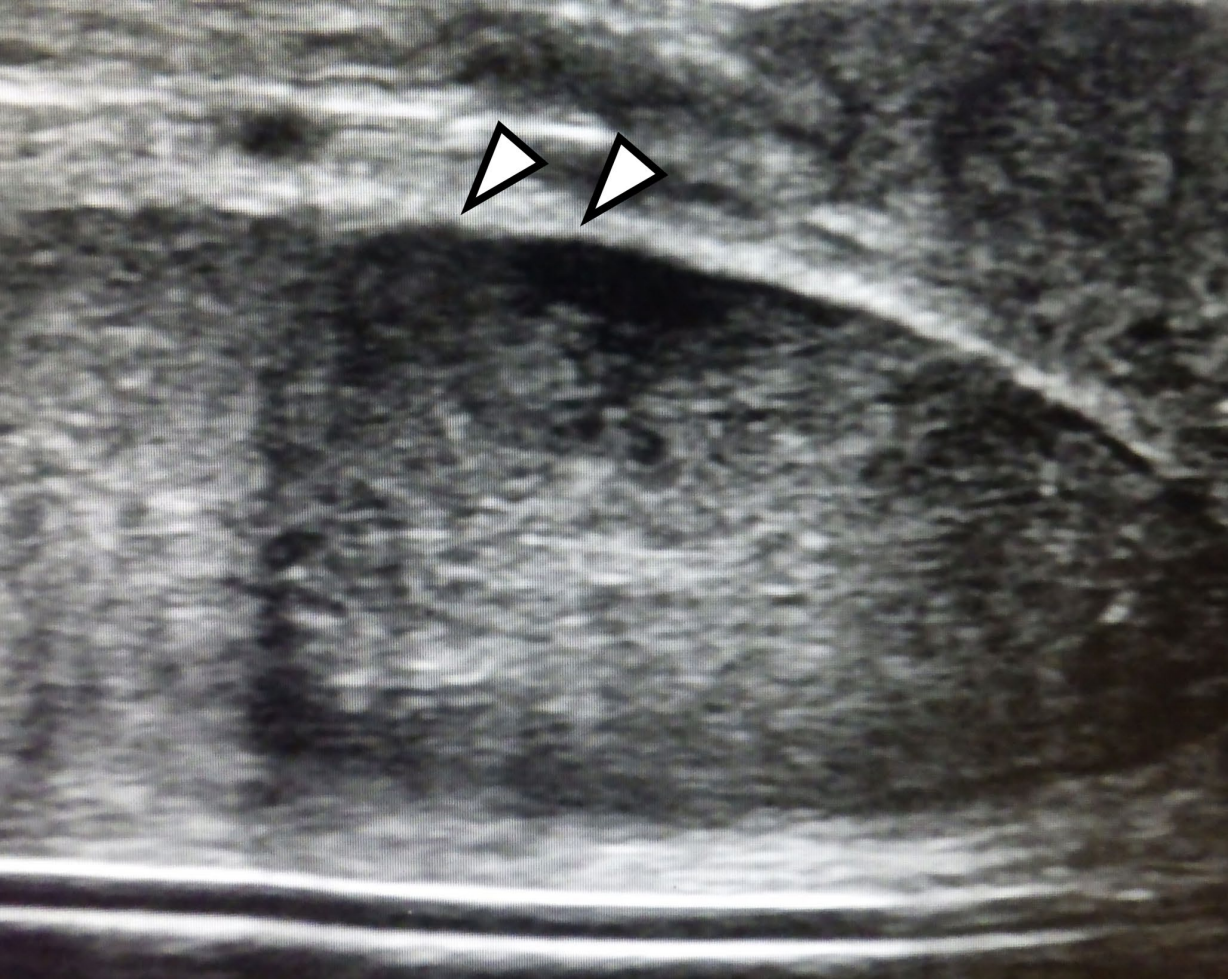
Ultrasonography revealed the white tone indurated lesion at the glans as a hypoechoic area (arrowhead)
